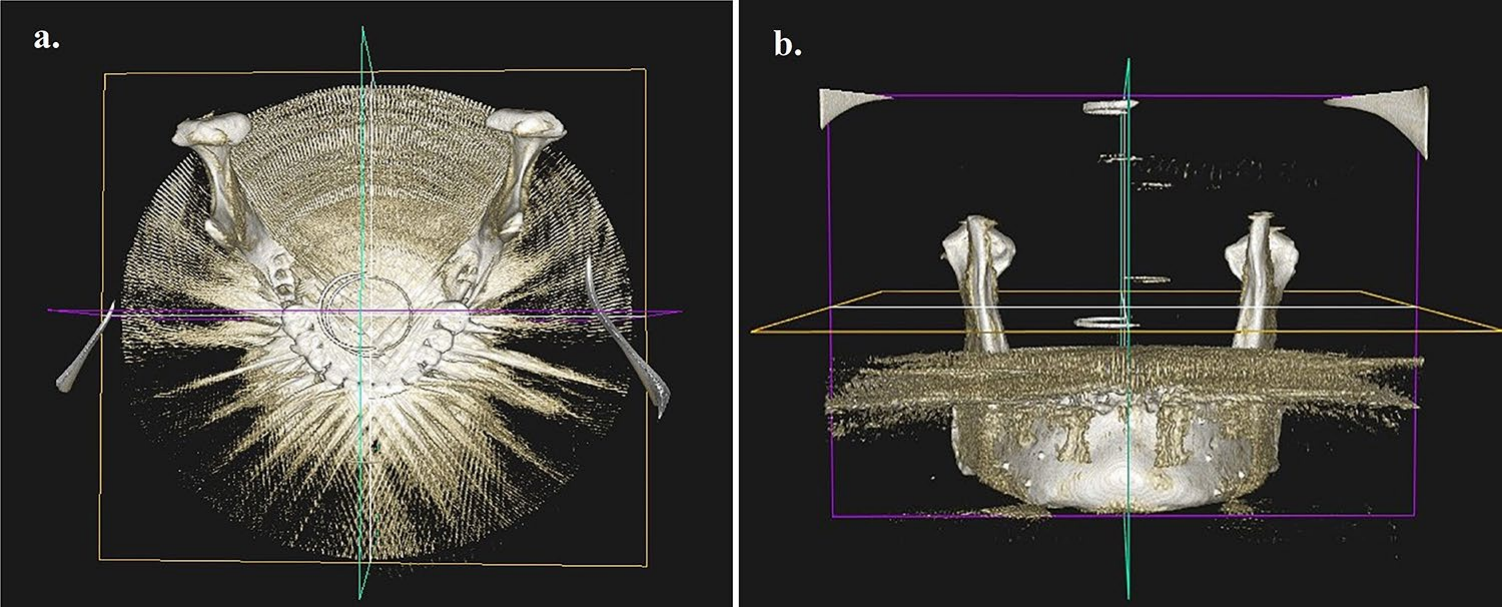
3-D CBCT image showing artifacts with implants at 33, 36, 43, and 46 and full arch prosthesis. (a) Occlusal view. (b) Frontal view.
